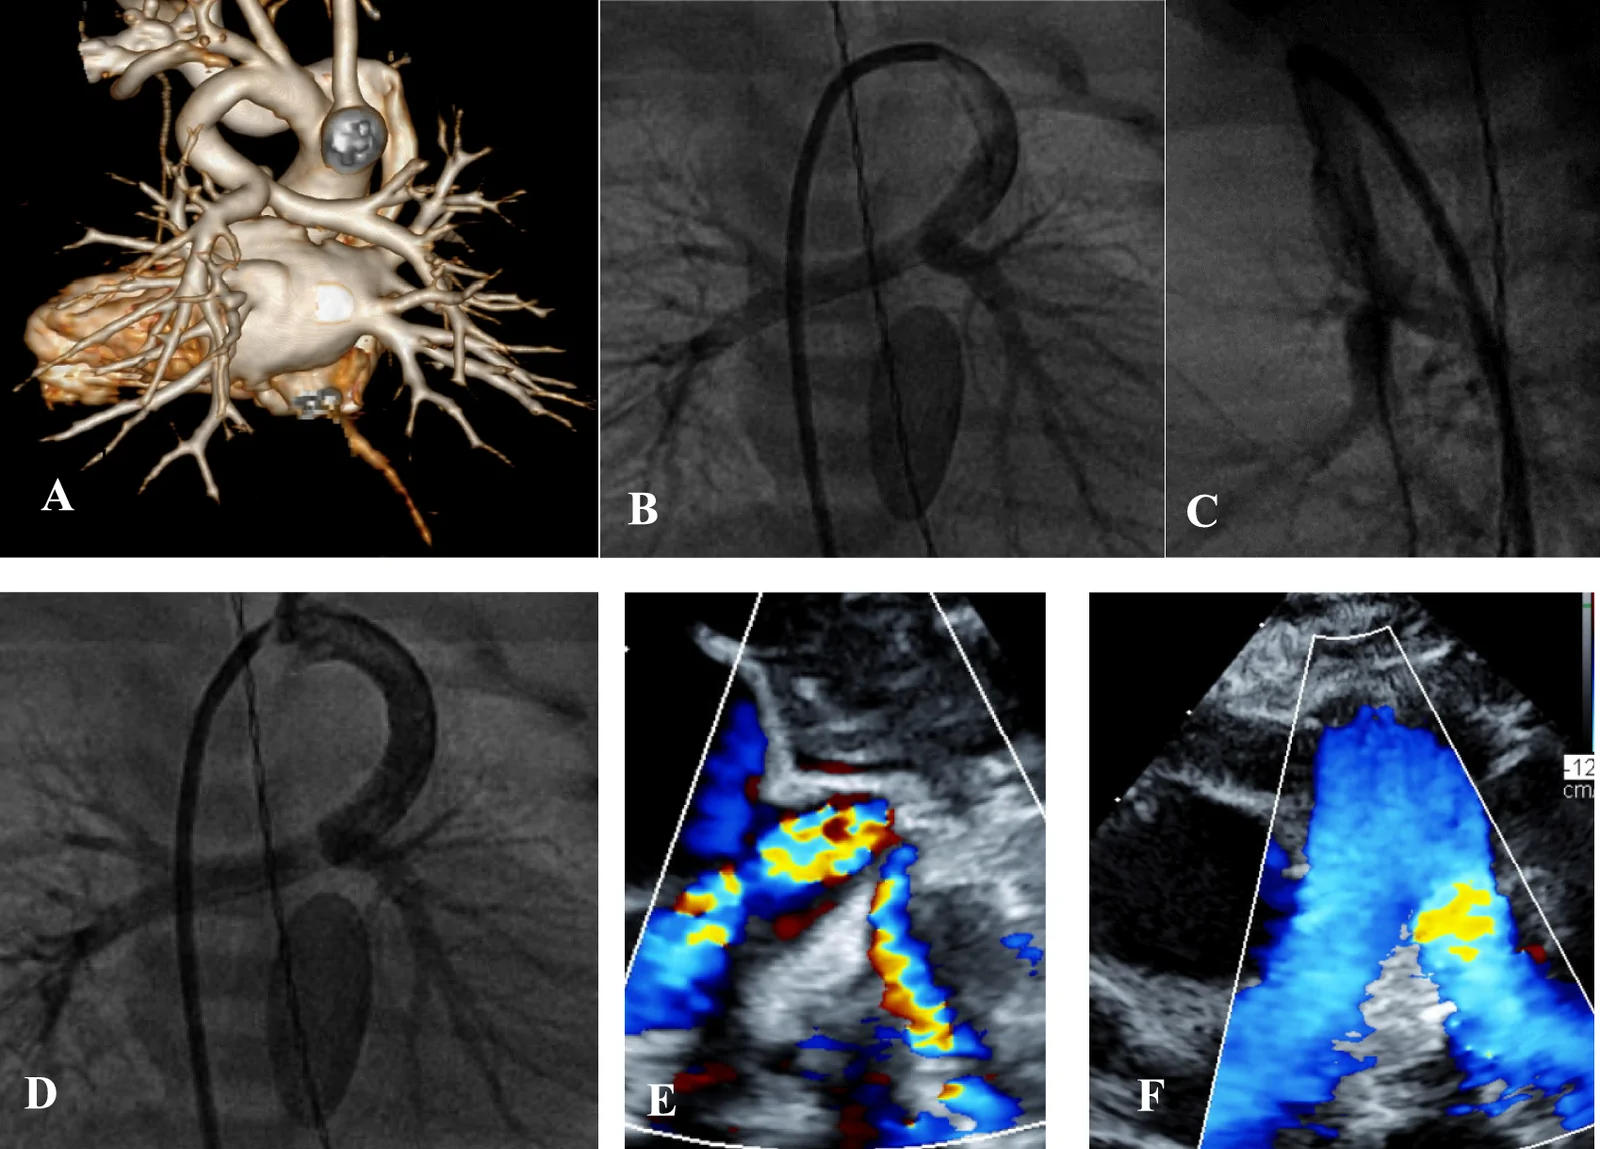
A 3D rendering of CT scan posterior view showing anatomy of a large PDA from the underside of the innominate artery and mild right pulmonary artery stenosis in a neonate with Tetralogy of Fallot, pulmonary atresia, and right aortic arch. B Angiography in anteroposterior view showing a large PDA supplying confluent branch pulmonary arteries and C Lateral view showing mild right pulmonary artery stenosis as seen on CT. D Angiography in anteroposterior and lateral views after PDA stenting with a 4*30 mm Onyx^TM^ Drug Eluting Stent, showing a well-seated stent and good flow to both pulmonary arteries. E Follow up echo six months after PDA stenting showing stenosis of the left pulmonary artery, and echo result F of no qualitative or quantitative pulmonary artery stenosis after Tetralogy of Fallot surgical repair with right ventricle to pulmonary artery homograft and pulmonary artery plasty
